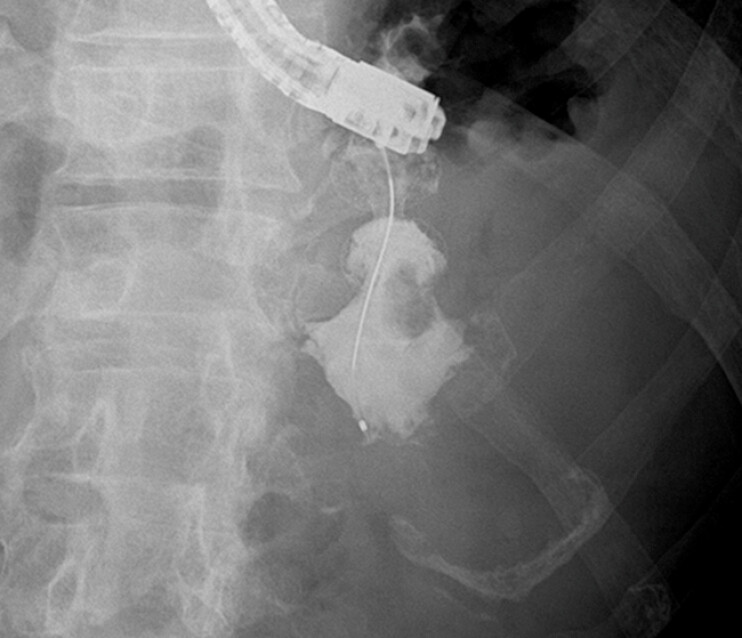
A radiographic image showing reduction of the walled-off necrosis and resolution of fistulas to the small intestine and the colon after lumen-apposing metal stent placement.
